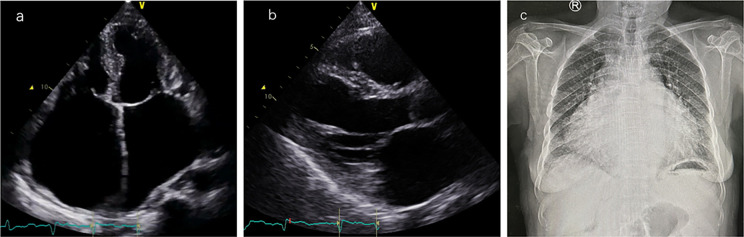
Cardiac imaging findings. (a) Apical four-chamber view: the left atrium has a vertical diameter of 8.9 cm and a transverse diameter of 5.9 cm; the right atrium has a vertical diameter of 9.2 cm and a transverse diameter of 6.6 cm. (b) Left ventricular long-axis view: left ventricular end-diastolic diameter (LVEDD) is 5.8 cm, left ventricular posterior wall thickness (LVPWT) is 1.1 cm, interventricular septal thickness (IVS) is 1.0 cm, and right ventricular anteroposterior diameter is 2.4 cm. (c) Anteroposterior chest x-ray showed significant heart enlargement.
